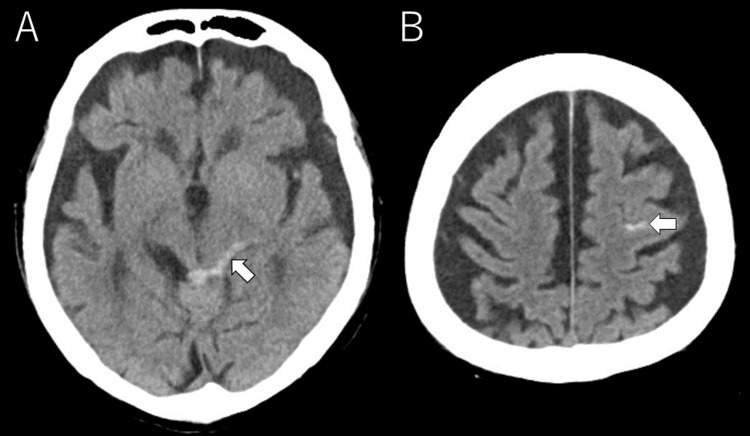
Initial head CT showing traumatic subarachnoid hemorrhage. (A, B) Axial non-contrast head CT images showing traumatic subarachnoid hemorrhage (arrows).
